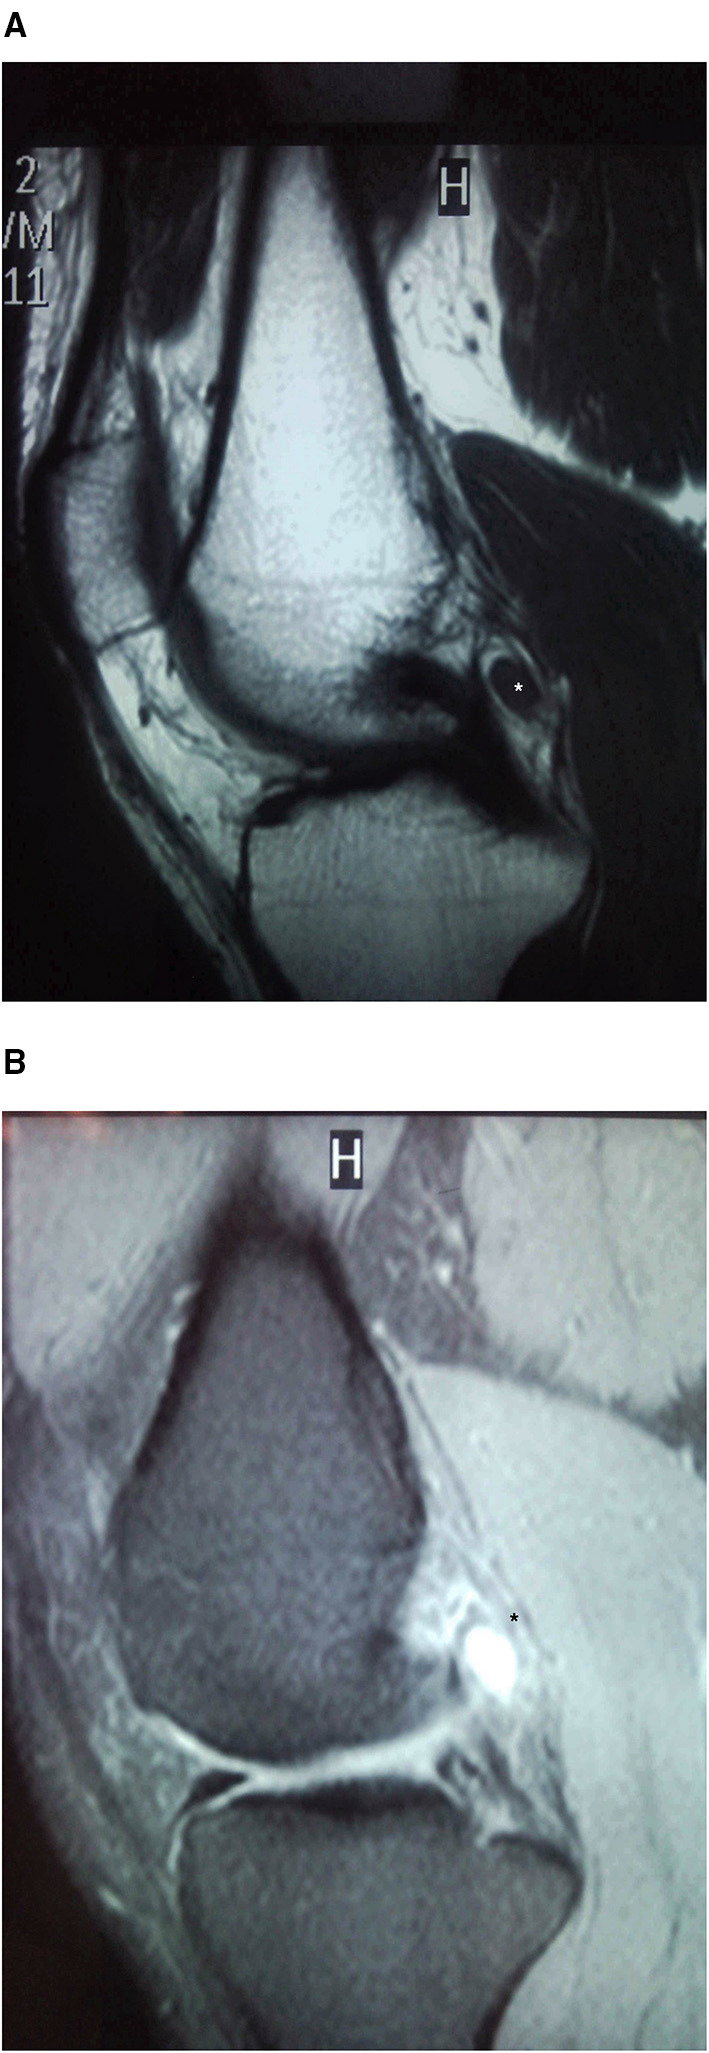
Case 4: posterior compartment SH MRI. Sagittal MRI sequences showing a well-defined intra-articular synovial mass in the posterior compartment of the knee, behind the PCL. On T1-weighted (A) sequences, the mass was isointense to the surrounding muscle with peripheral heterogeneous areas; the mass was hyperintense on T2-weighted images (B). *Location of the Synovial Haemangioma tumor in the knee.
